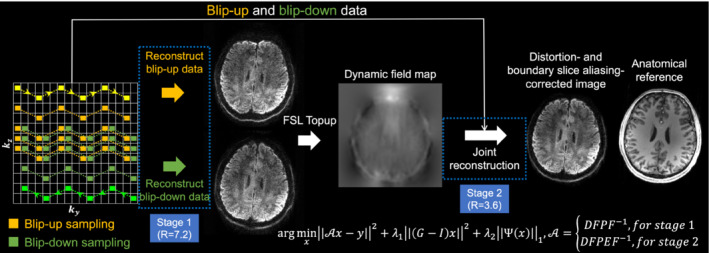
Proposed framework. The proposed sampling pattern with k_z_ blipped‐CAIPI and complementary partial Fourier for blip‐up (yellow) and blip‐down (green) data and the two‐stage reconstruction. The trajectory of one shot of the multi‐shot sampling is marked in bright yellow and bright green for blip‐up and blip‐down sampling with arrows indicating the phase encoding direction. The forward operator A in the reconstruction includes Fourier transform F and inverse Fourier transform F^‐1^, the phase modulation P representing motion‐induced phase errors measured by 2D navigators, k‐space sampling operation D in both stages 1 and 2, and an additional distortion operation E (captured by the field map) for stage 2. Example images are from a single volume diffusion MRI dataset (1.05 mm isotropic resolution) of a representative subject, with anatomical image listed for reference (acquired with MPRAGE at 0.86 mm isotropic resolution).
